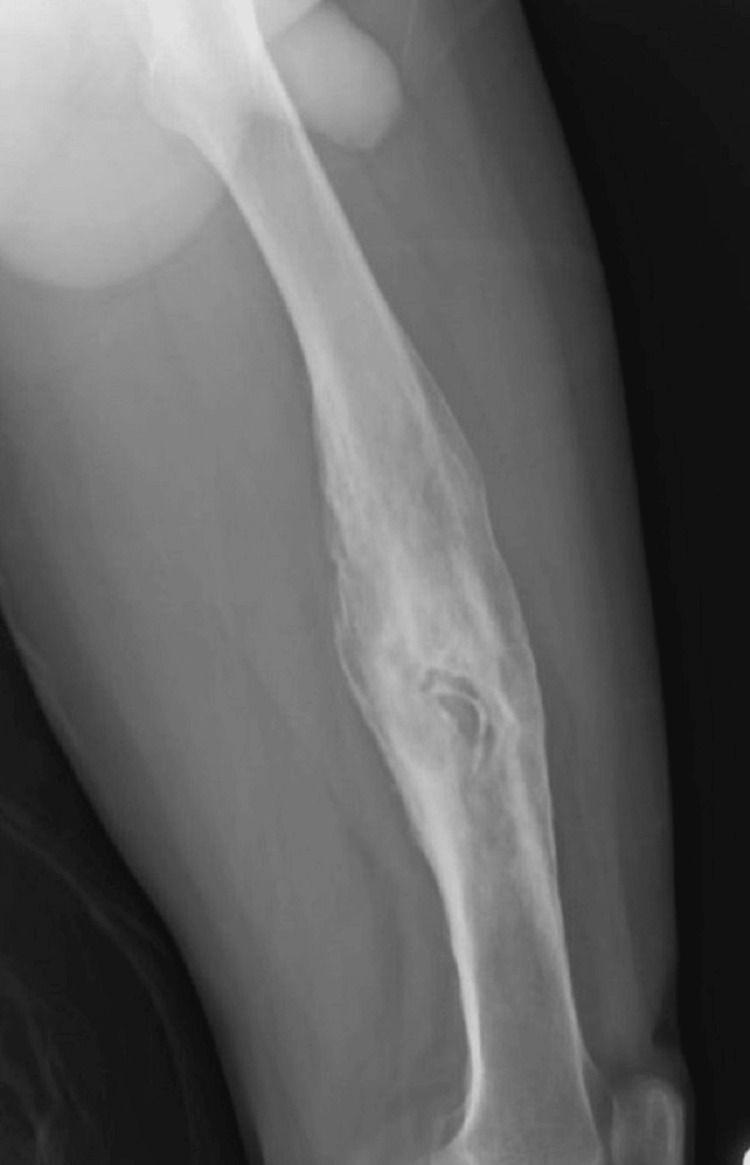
Preoperative radiograph showing the sequestrum surrounded by sclerotic bone
